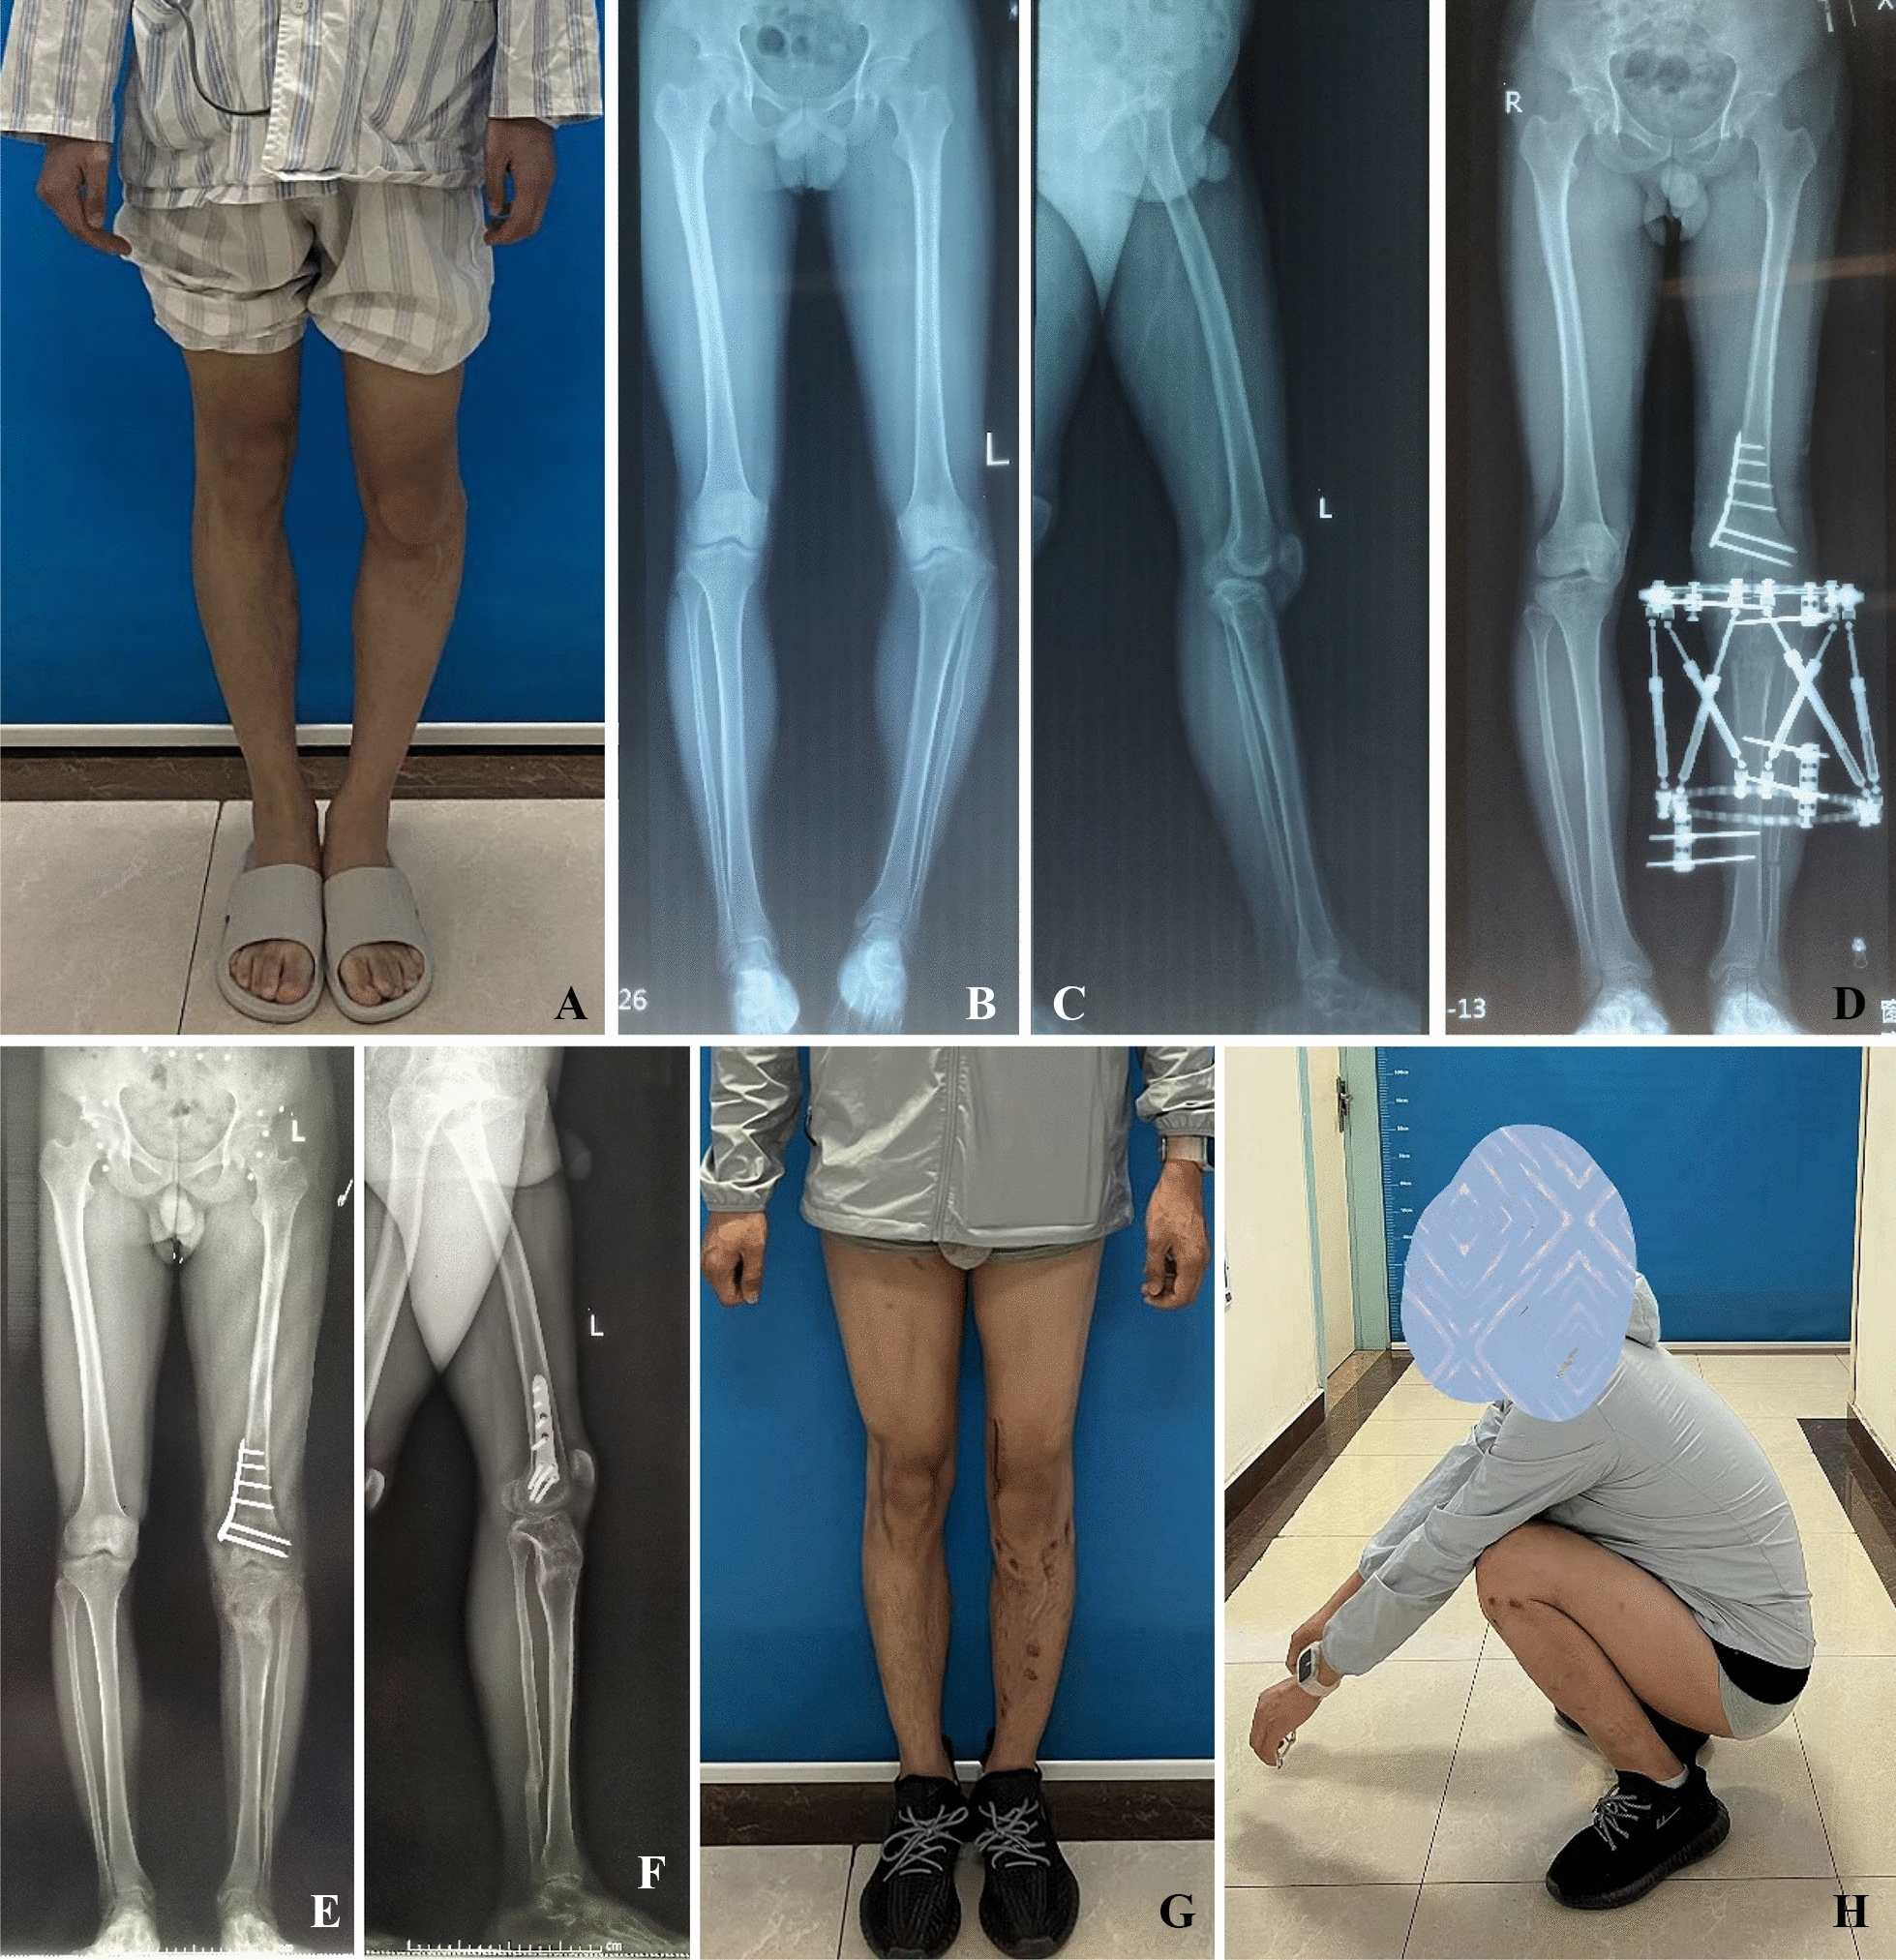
A 28-year-old male with juvenile Blount disease underwent osteotomy at the age of 14, and the deformity recurred 2 years later. A, B and C Preoperative appearance and radiograph of lower limbs. D One-stage left tibial osteotomy QSF fixation and distal femoral osteotomy with internal fixation. The external frame was adjusted for 45 days, and the osteotomy site healed 10 weeks after the adjustment. At this point, the external fixator was removed. E, F, G and H Appearance and radiograph of the patient's lower extremities after the frames were removed
